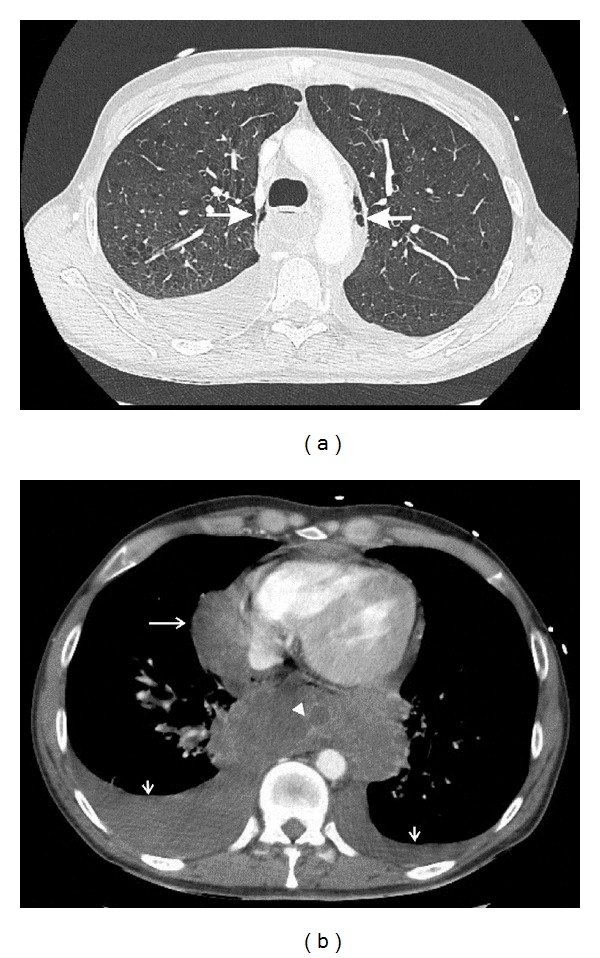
Contrast-enhanced CT scan of the thorax: (a) lung window, confirming pneumomediastinum (closed arrows), and (b) mediastinal window showing a tear in the right posterolateral wall of the distal oesophagus (arrowhead), bilateral pleural effusions (short arrows), massive retrocardiac collections, and a paracardial collection (open arrow) corresponding with the silhouette sign on chest X-ray.
